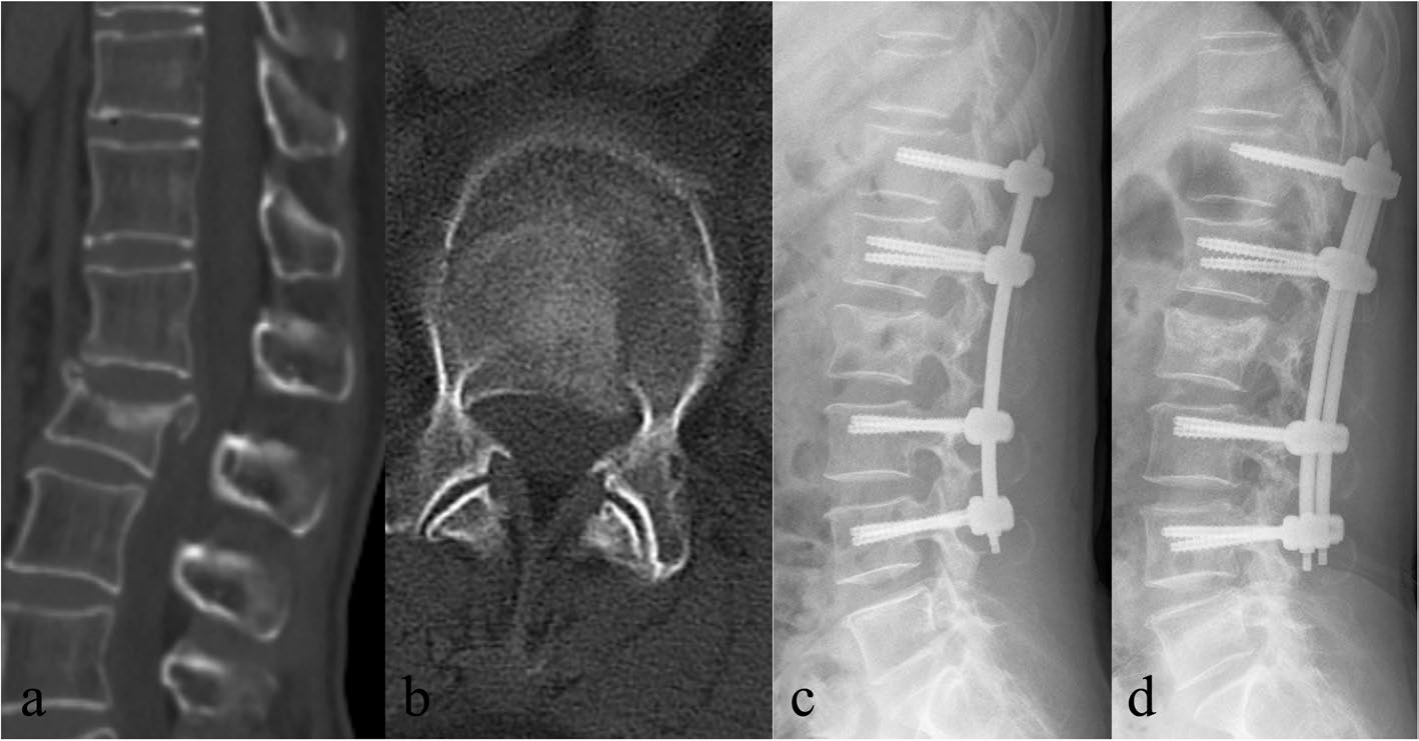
a-b Preoperative Sagittal and axial CT images of a 62-year-old female showing L2 burst fracture; c Immediate postoperative lateral plain radiograph showing AVH and kyphotic deformity were corrected after LF treatment; d Plain radiograph at the final follow-up
